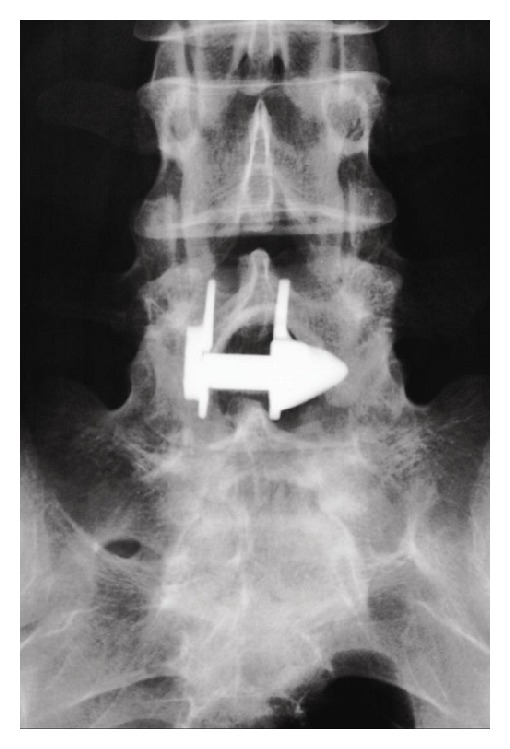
Anterior-posterior X-ray image showing an X-Stop device implanted at L4-L5 interspinous level.
